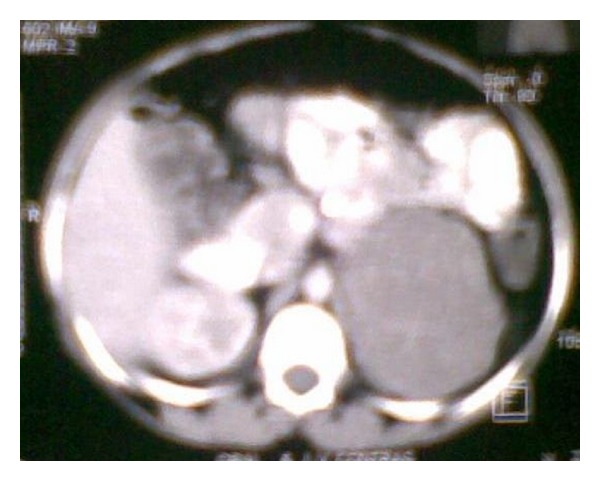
Enlarged view of CT scan image in cross section, showing a large well-circumscribed mildly enhancing mass having few calcification at left suprarenal region.
